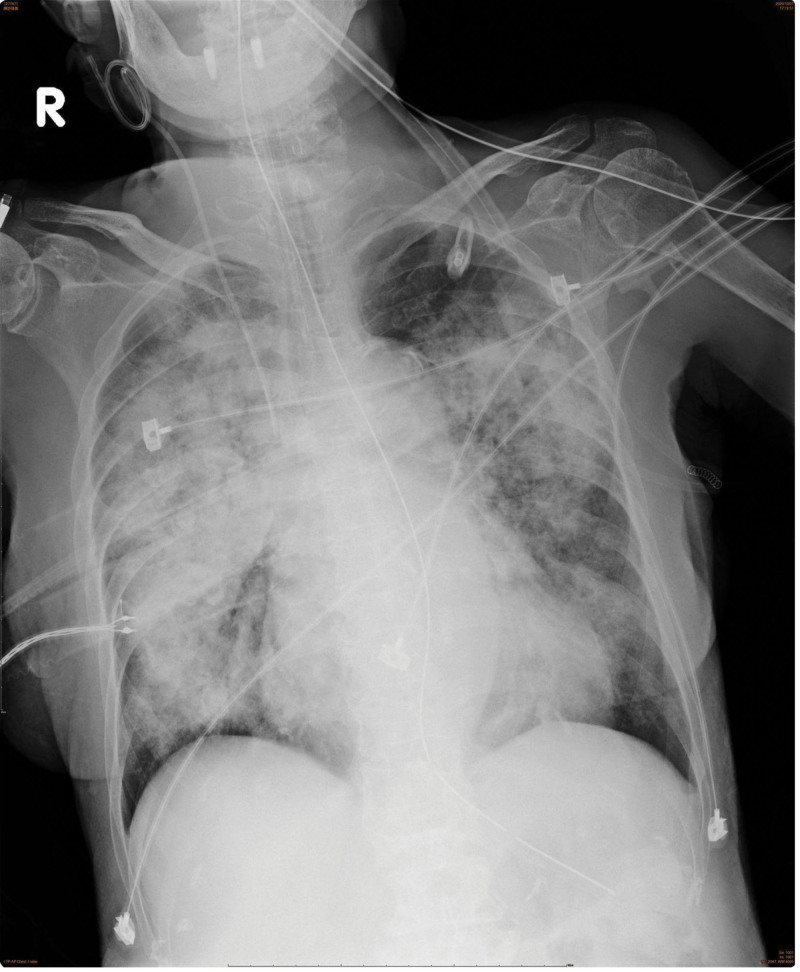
Contrast-enhanced chest CT showing an enlarged main pulmonary artery and extensive bilateral lung consolidations, initially interpreted as pulmonary edema or pneumonia. CT = computed tomography.
